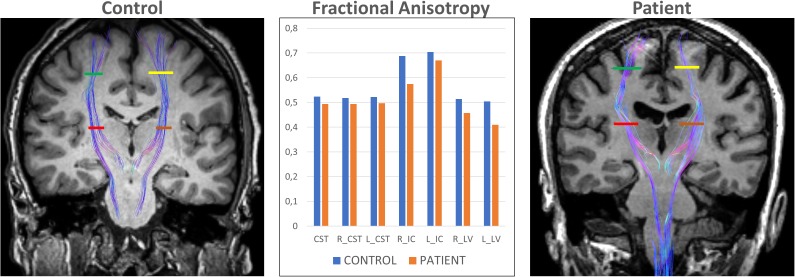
DTI tractography overlaid on coronal T1-weighted images of a control subject (left) and exemplary ELA patient (right) evaluated in our center. The Corticospinal Tract (CST) at 2 different levels allows comparing the differences in Fractional Anisotropy between control subjects and ELA patients. R_CST, right CST; L_CST, left CST; R_IC, right Posterior Limb of Internal Capsule (red line); L_IC, left Posterior Limb of Internal Capsule (brown line); R_LV, right Centrum Semiovale at top of the lateral ventricle (green line); and L_LV, left Centrum Semiovale at top of the lateral ventricle (yellow line).
